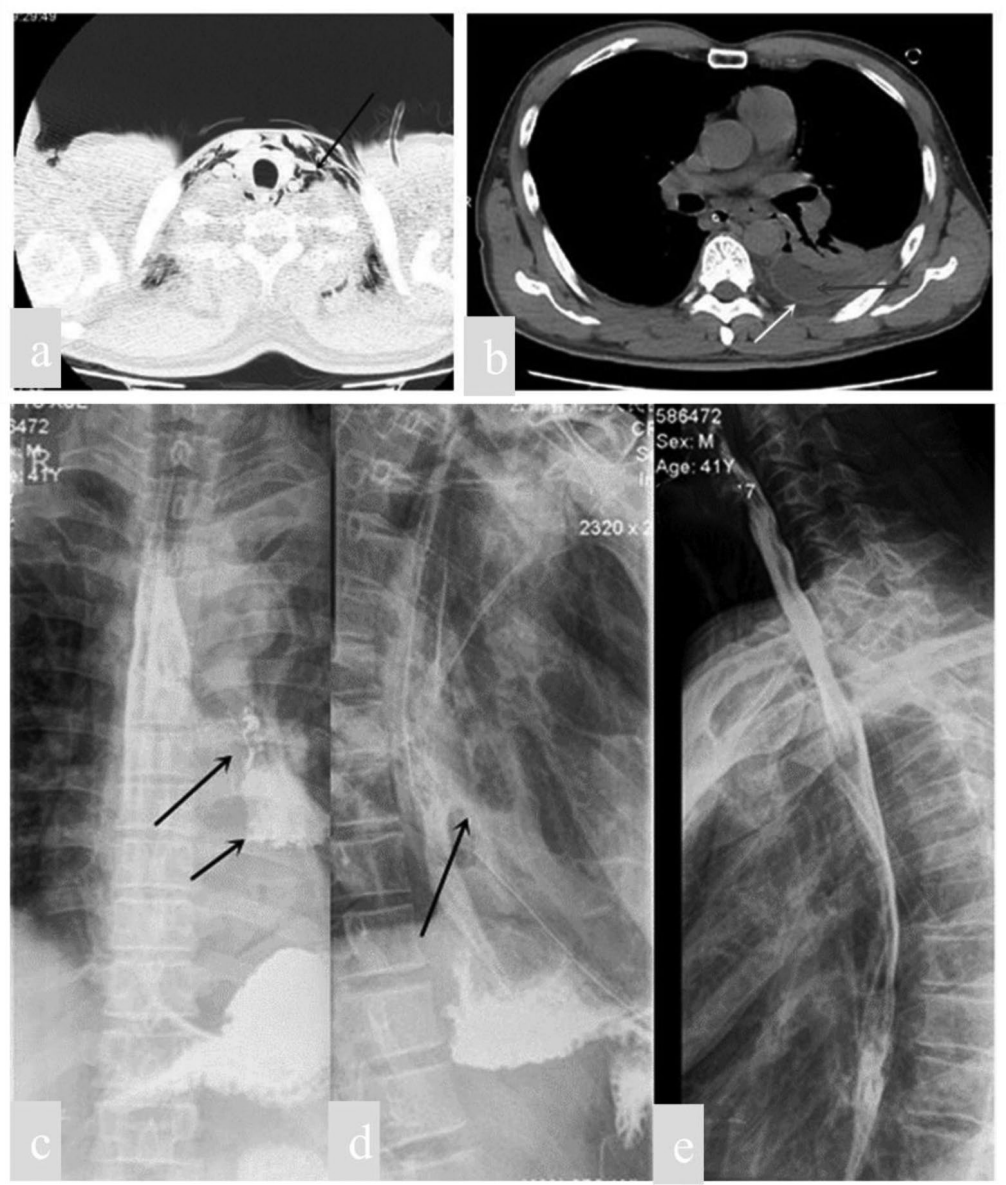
CT scan of the chest revealed subcutaneous and mediastinal emphysema in the chest and left Encapsulated Effusion. Esophagography showed an extensive tear on the left side of the middle and lower portions of thoracic esophagus. a Lung window, Arrow: subcutaneous and mediastinal emphysemas. b Mediastinal window, Arrow: encapsulated effusion. c Postero-anterior position, d left lateral position, e right lateral position. Arrow: contrast agent entered into left thoracic cavity through the ruptured esophagus
